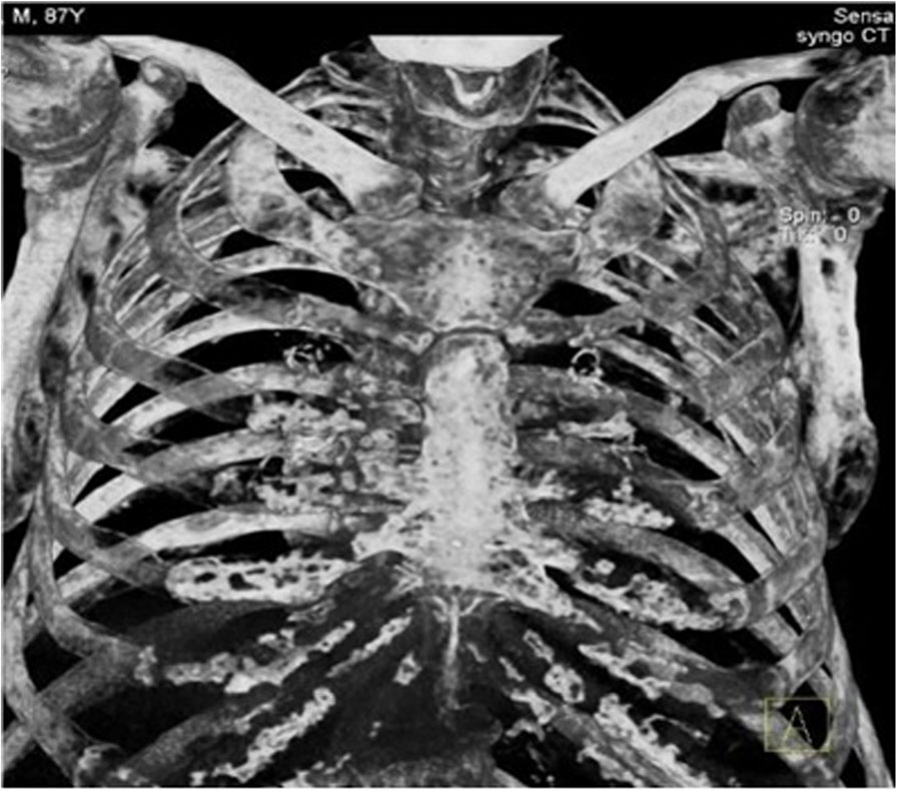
Chest computed tomography scan. Severe lytic lesions involving several costal ribs
